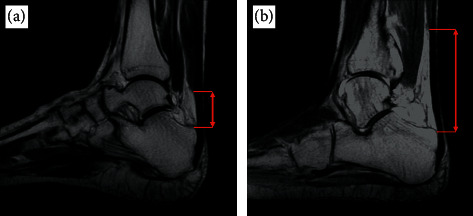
Free Achilles tendon length differences on sagittal T1-weighted MR images. (a) A patient with a short free Achilles tendon (short red arrow). (b) A patient with a long free Achilles tendon (long red arrow).
